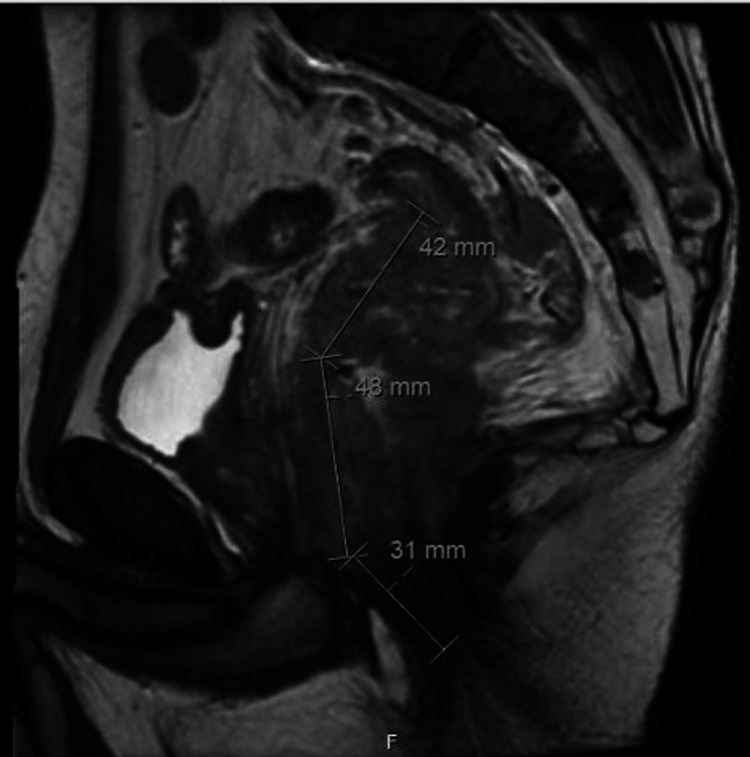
Saggital thin slice, fast-recovery fast spin-echo T2-weighted MRI MRI of the pelvis with contrast: multiplanar T2-weighted, diffusion, and dynamic. Post-gadolinium images were obtained. IMPRESSIONS:
